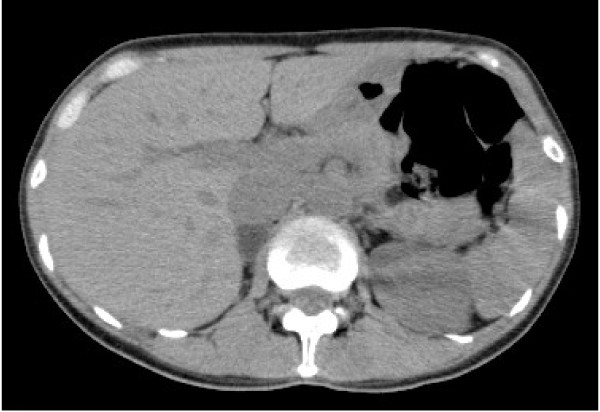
Abdominal computed tomography showing a 19mm right adrenal tumor.
